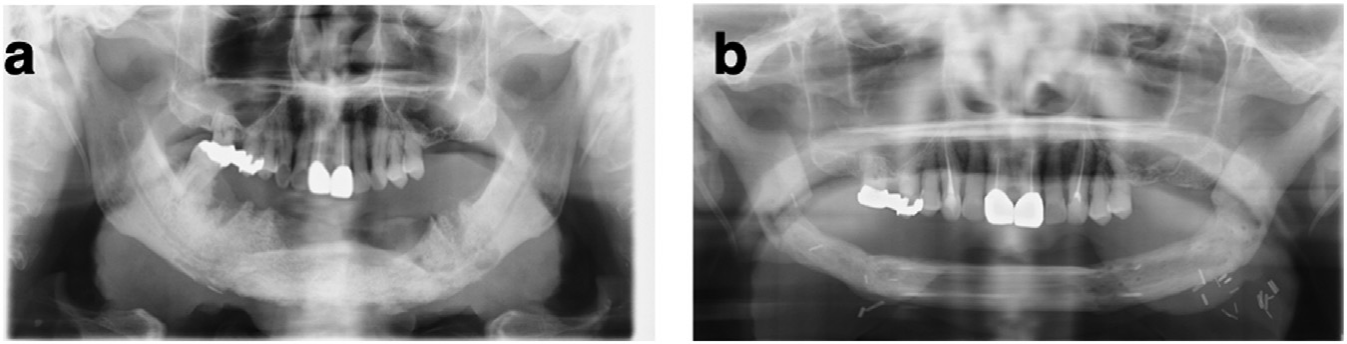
Clinical photographs showing (a) Preoperative radiograph of the face. (b) Postoperative radiograph of the face.
